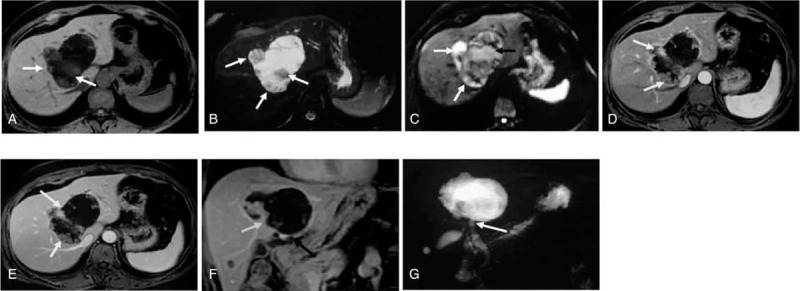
Magnetic resonance imaging of a 51yr old female patient with intrahepatic ductal papillary neoplasm with infiltrating carcinoma. (A) T1 transaxial section. (B) T2 transaxial section. Multiple nodules of inner wall showed T1 hypointense and T2 hyperintense relative to normal hepatic parenchyma (white arrows). Fluid inside the lesion showed signal as water. (C) DWI indicated multiple nodules (white arrows) and fluid inside the lesion (black arrow) with hyper-signal. (D) Arterial phase transaxial section. (E) Venous phase transaxial section. (F) Delayed-phase coronal section. The enhanced manifestation of multiple nodules was similar to CT (white arrows). The lower edge involved the hepatic capsule mildly (black arrow). (G) MRCP indicated a giant spherical lesion of hyper-signal communicated with the ectatic intrahepatic bile duct (white arrow). DWI = diffusion weighted images, MRCP = magnetic resonance cholangiopancreatography.
